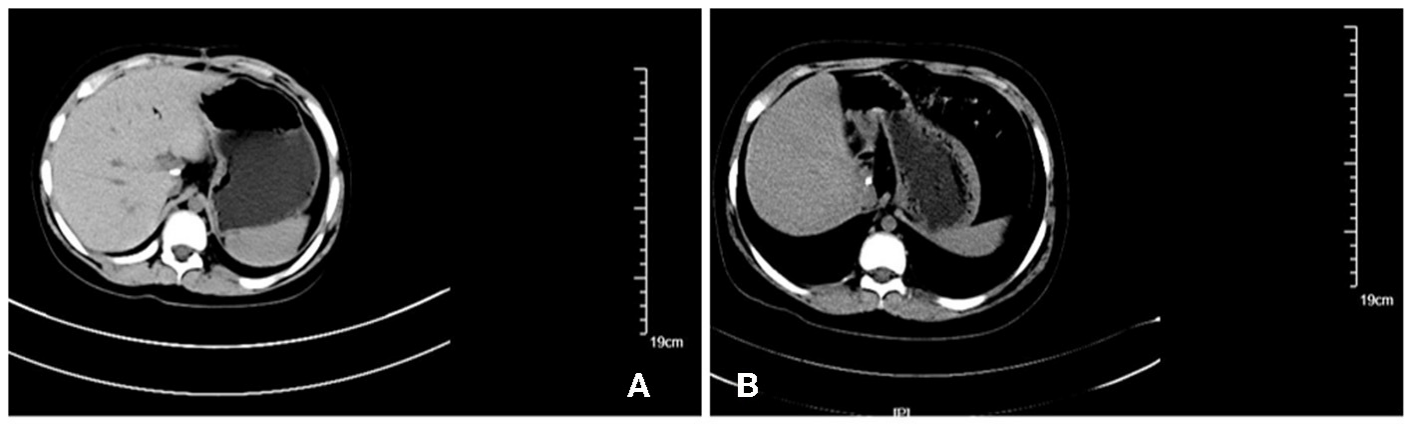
Imaging findings during follow up: there was no abnormal density shadow in the liver, which was consistent with the changes of hepatic caudate lobe resection. (A) abdominal computed tomography of the abdomen 2 months after the surgery; (B) Enhanced computed tomography of the abdomen 14 months after the surgery.
